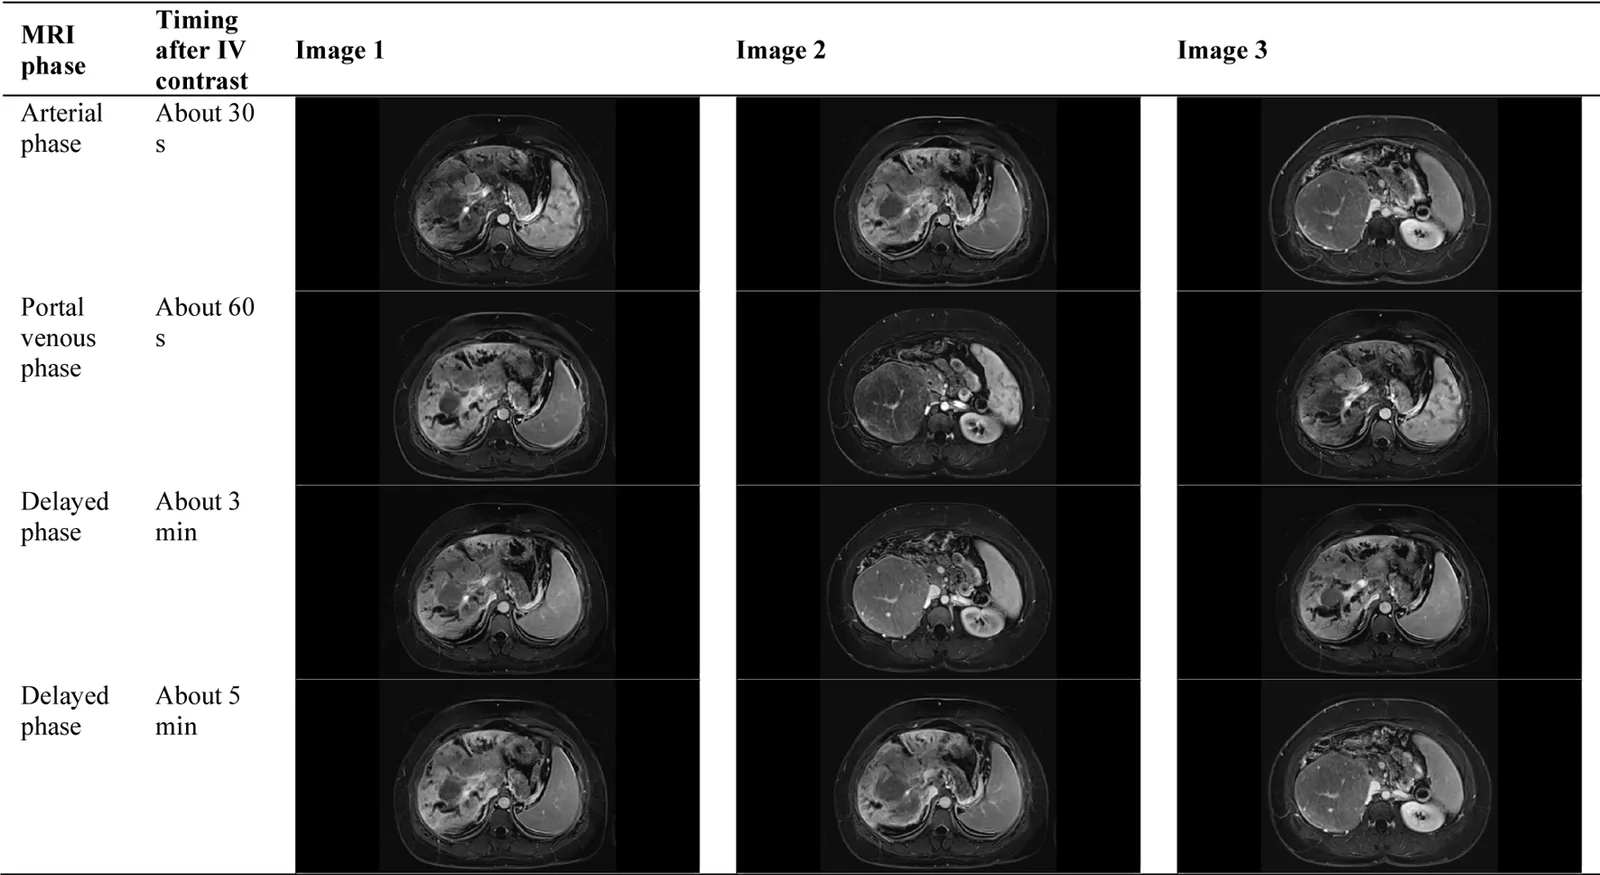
Triphasic Contrast-Enhanced MRI of the Liver Demonstrating Enhancement Characteristics of FNH With Patent Arterial/Venous Flow Through All Phases of Scan. MRI: magnetic resonance imaging; FNH: focal nodular hyperplasia; IV: intravenous.
